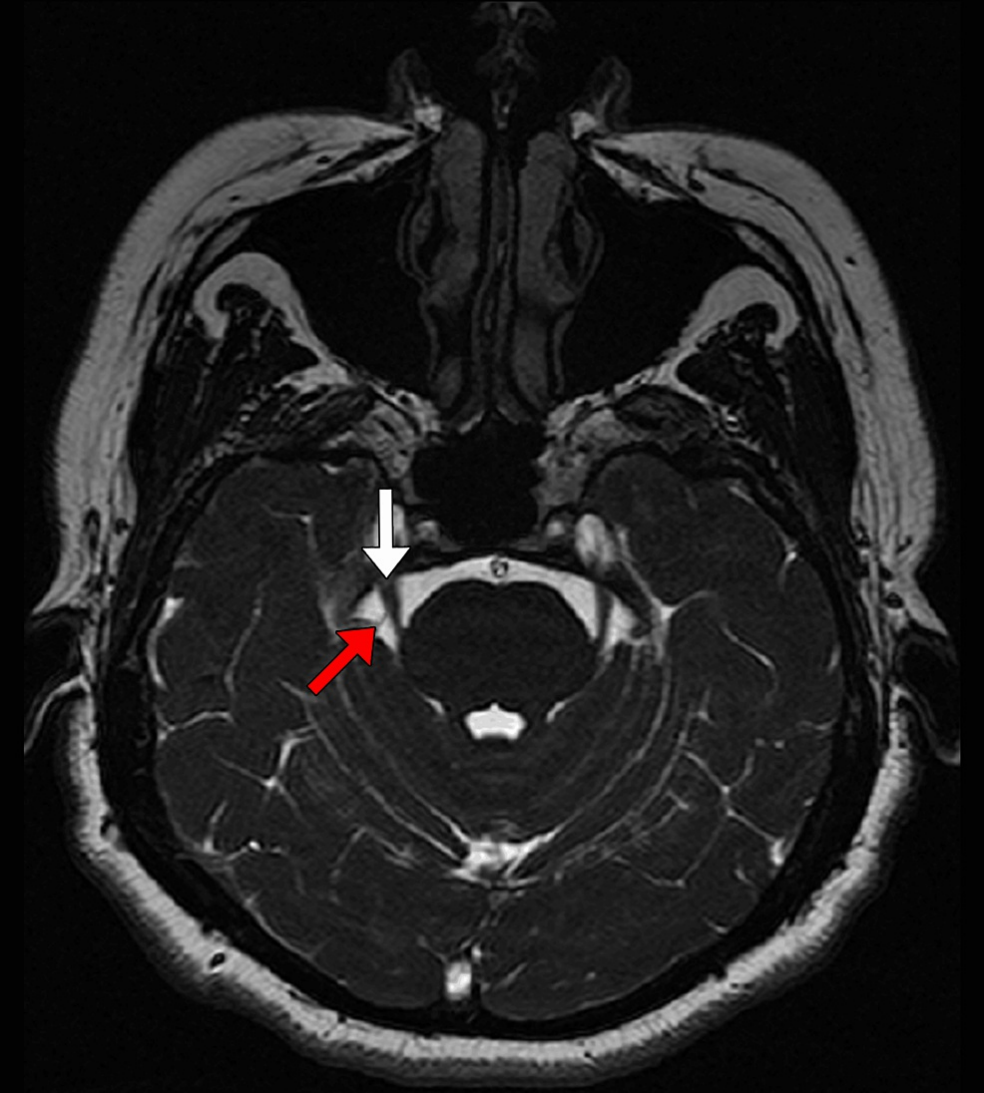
3D-FIESTA MRI showing a false negative for vascular conflict involving the CN V The white arrow indicates the right fifth cranial nerve (CN V) in its cisternal path, and the red arrow indicates a possible culprit vessel. The MRI corresponds to patient one. FIESTA - fast imaging employing steady-state acquisition
